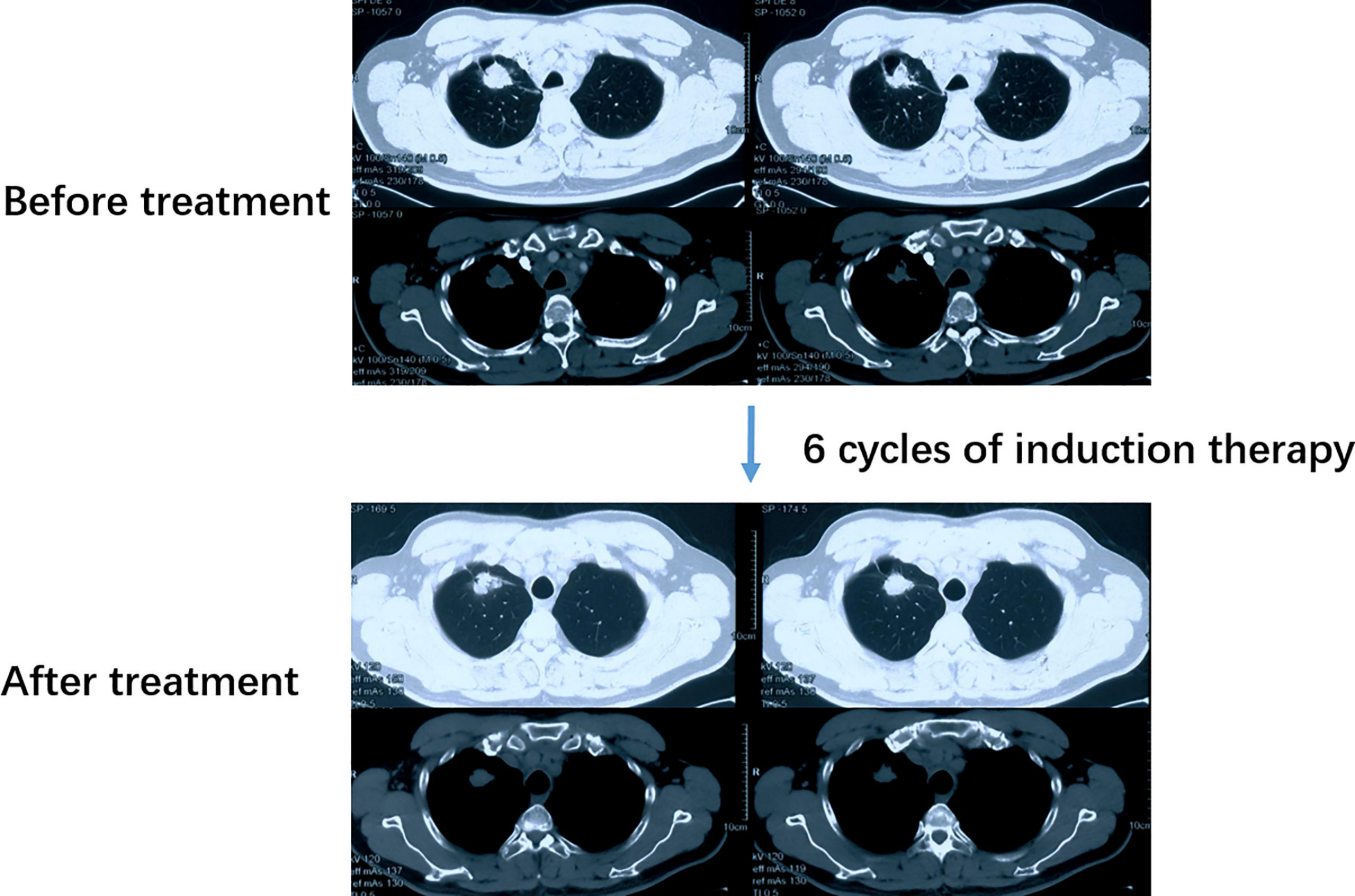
CT scan of the patient before and after induction therapy showed the tumor response. CT, Computed tomography.
